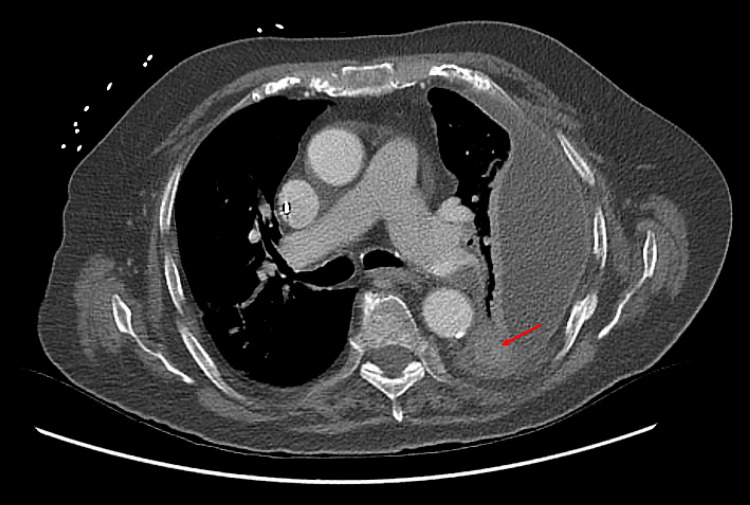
Computed tomography of the chest demonstrating calcifications in the left lower lobe of the lung, suggestive of microaspirations (red arrow)
